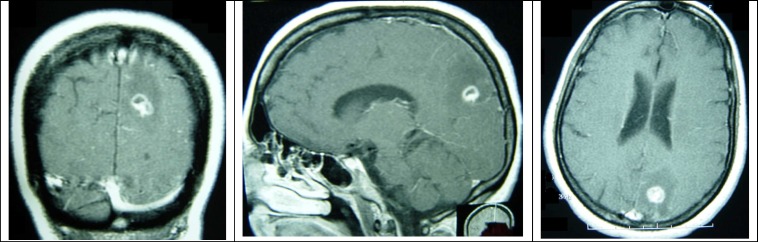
T_1_-weighted magnetic resonance imaging with gadolinium enhancement shows a single 1.5 cm targetoid lesion with ring enhancement and parenchymal vasogenic edema surrounding the lesion in the left posterior parietal lobe
